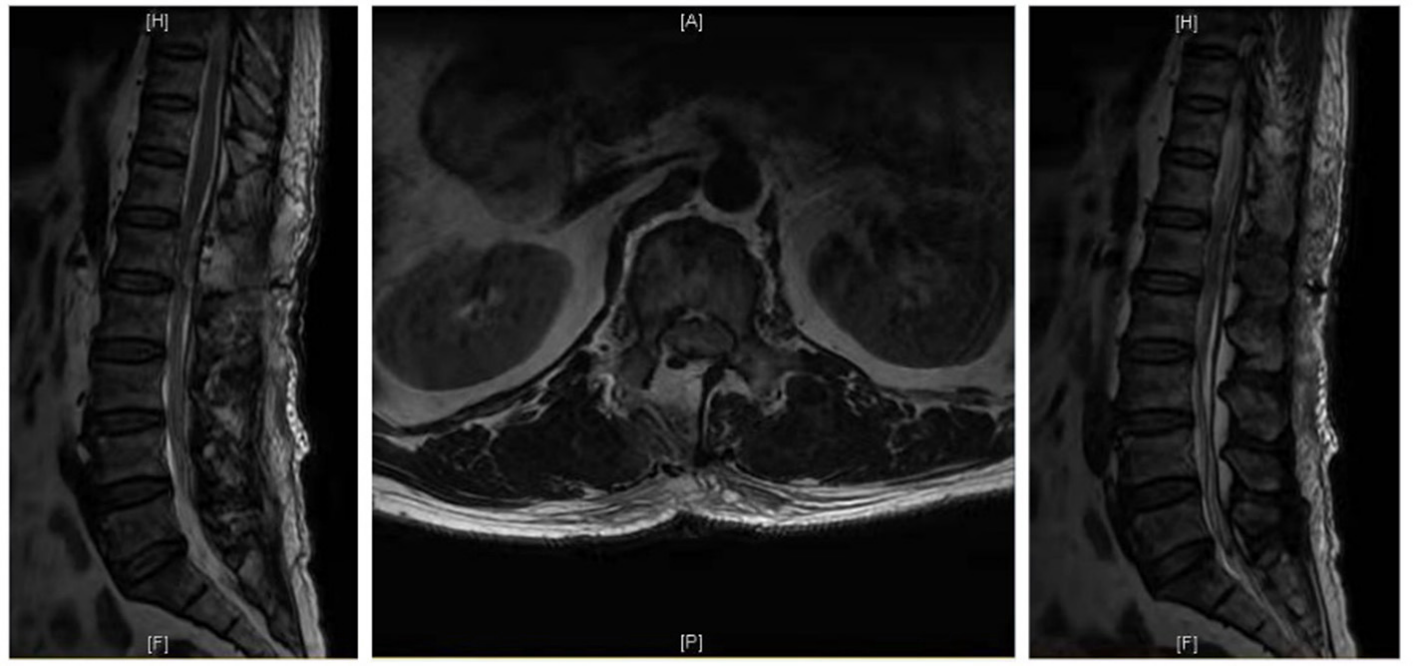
Postoperative MRI of the case 1.
